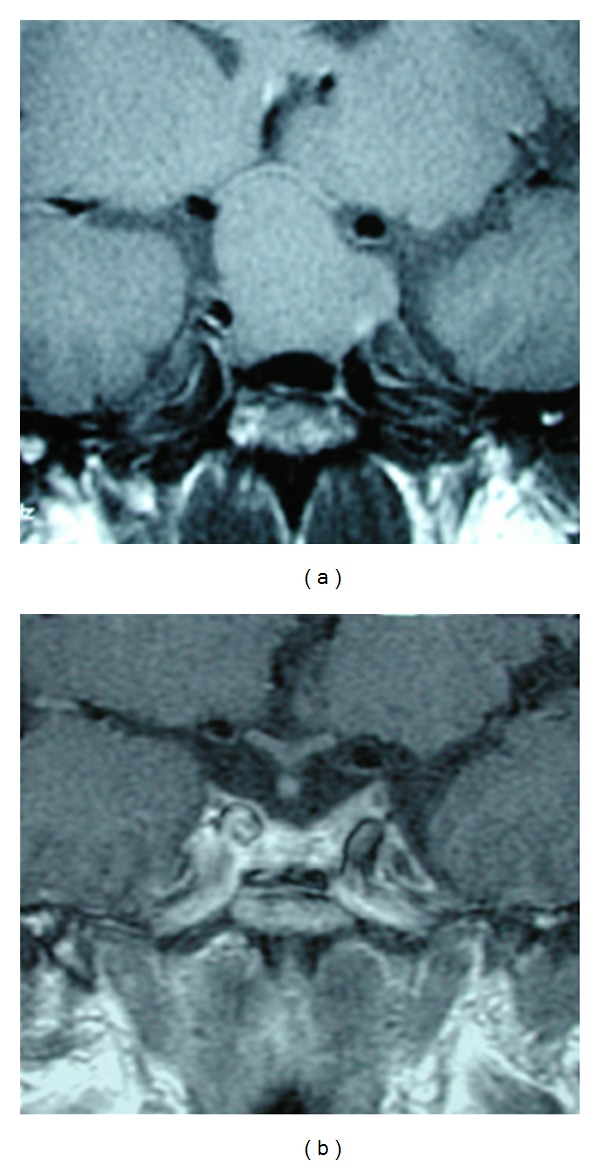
Enhanced MRI coronal view that shows a tumor which is only displacing (but not invading) the left CS (a). On postoperative control, imaging (b) is possible to observe that the whole tumor was macroscopically removed. TS approach was used because the CS was not really invaded.
